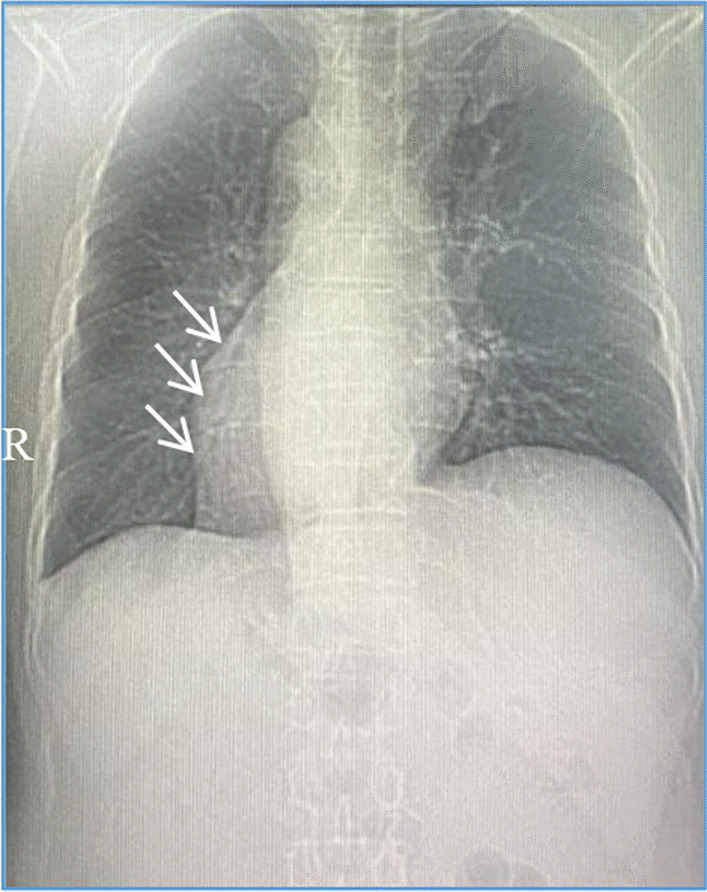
Preoperative digital radiography (DR): Demonstrates situs inversus. The position pointed to by the arrow is the left margin of the heart
